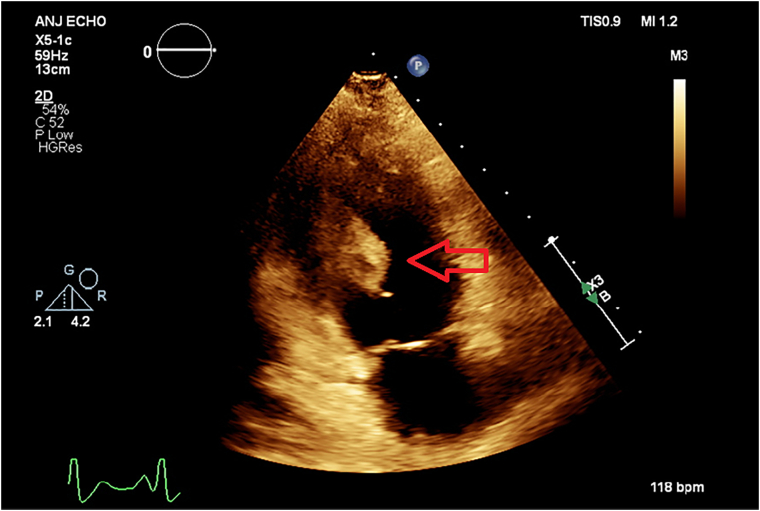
Two-chamber transthoracic echocardiography showing the thrombus.
